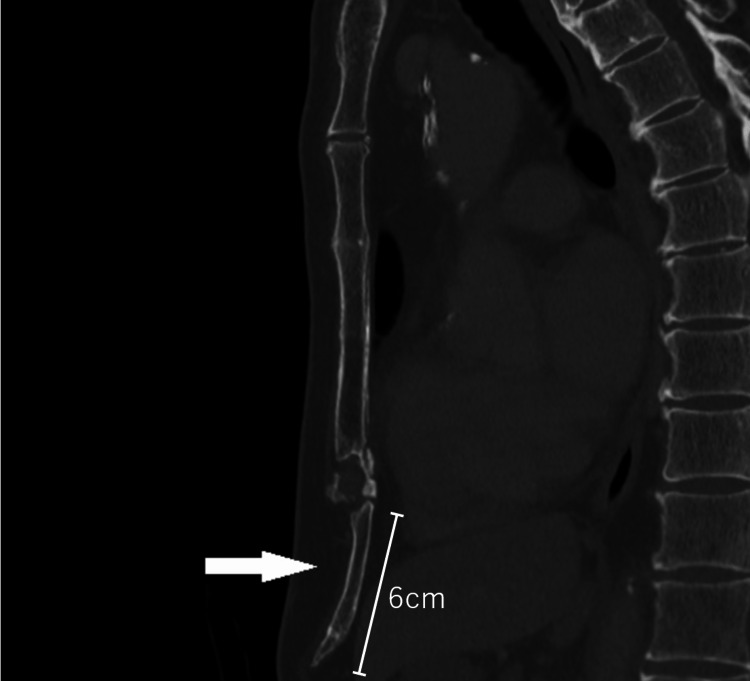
Large xiphoid process (white arrow) seen on CT
